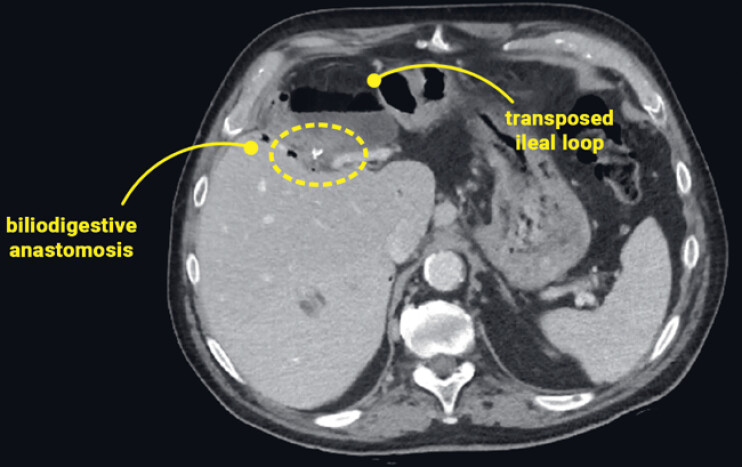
A preoperative abdominal CT scan showing the dilation of the afferent tract of the transposed loop with suspected impaired biliodigestive anastomosis discharge. CT, computed tomography.
